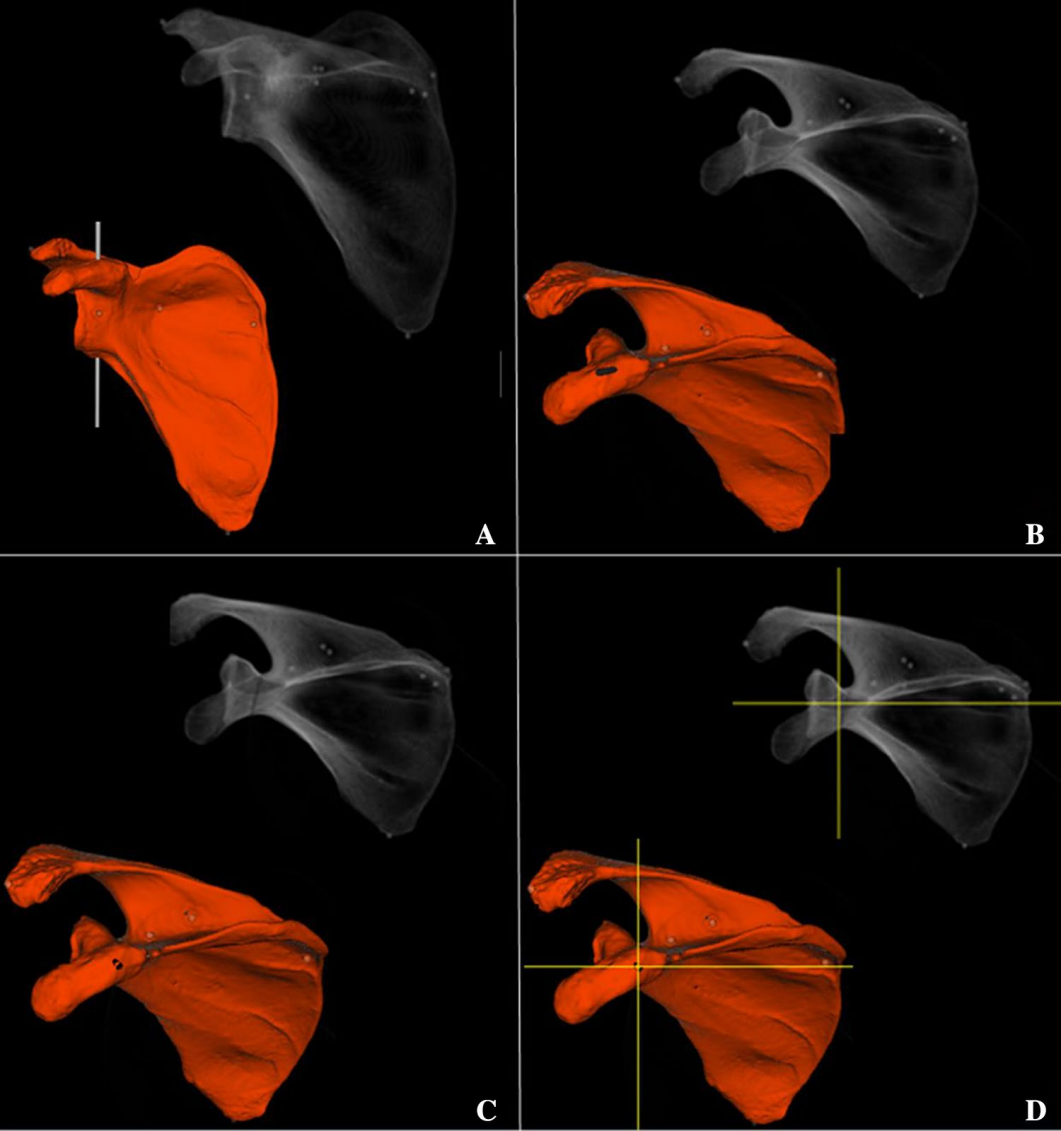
CTV protocol for fluoroscopy imaging: a view scapula in radiographic anterior–posterior view: identify the glenoid fossa, coracoid, acromion, scapular notch, superior scapular border, medial border, inferior border and scapula spine; b align viewing angle in cephalad direction until oval shaped tunnel appears; c adjust viewing angle until glenoid fossa is parallel to drilling direction; d the crosshair marks the optimal screw position
